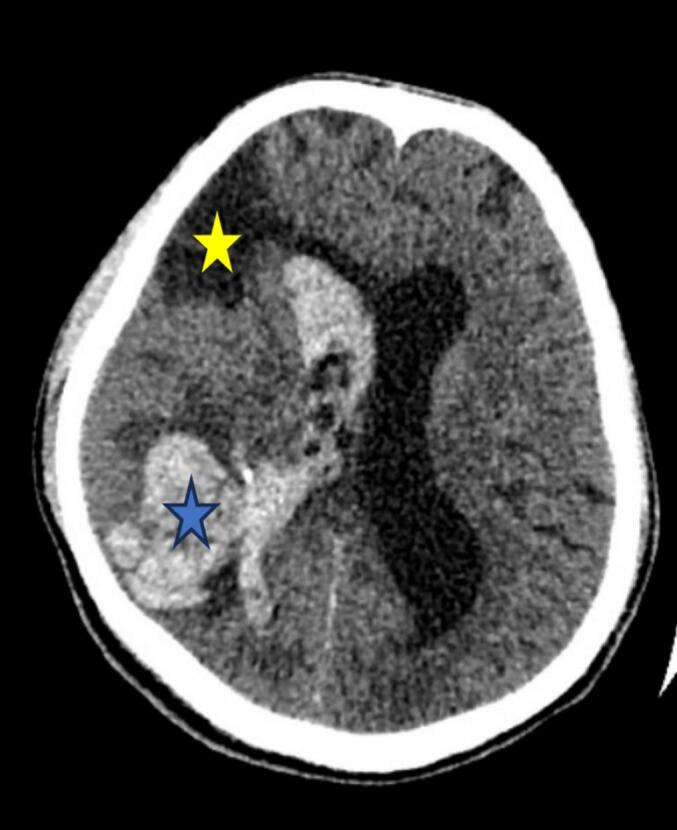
A non-contrast head CT scan showing right parietal ICH (blue star) with intra-ventricular extension (yellow star).
